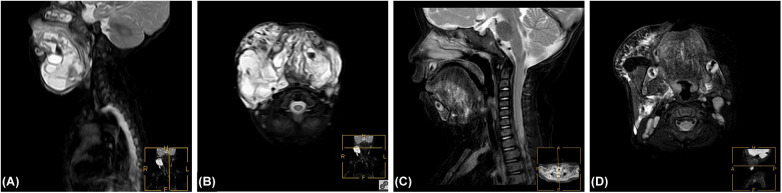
(A): Pre-treatment MRI sagittal fat-suppressed image shows a mixed-signal mass in the head and neck region, suggestive of a hemorrhagic lymphatic malformation. (B): Pre-treatment MRI axial fat-suppressed T2-weighted image reveals an irregular, heterogeneous hyperintense lesion in the head and neck with patchy hemorrhagic signal patterns. The lesion has indistinct margins and causes displacement of surrounding soft tissues. (C): Post-treatment sagittal fat-suppressed T2-weighted MRI after initial pingyangmycin and 1% lauromacrogol alternating sclerotherapy followed by 7 months of oral sirolimus adjuvant therapy shows significant volume reduction of the original lesion with more uniform signal intensity and markedly alleviated compression of surrounding tissues. (D): Post-treatment axial fat-suppressed T2-weighted MRI demonstrates a markedly reduced area of the original mixed-signal lesion with near-complete resolution of abnormal hyperintensity, revealing clear anatomical structures including surrounding muscles and vessels.
